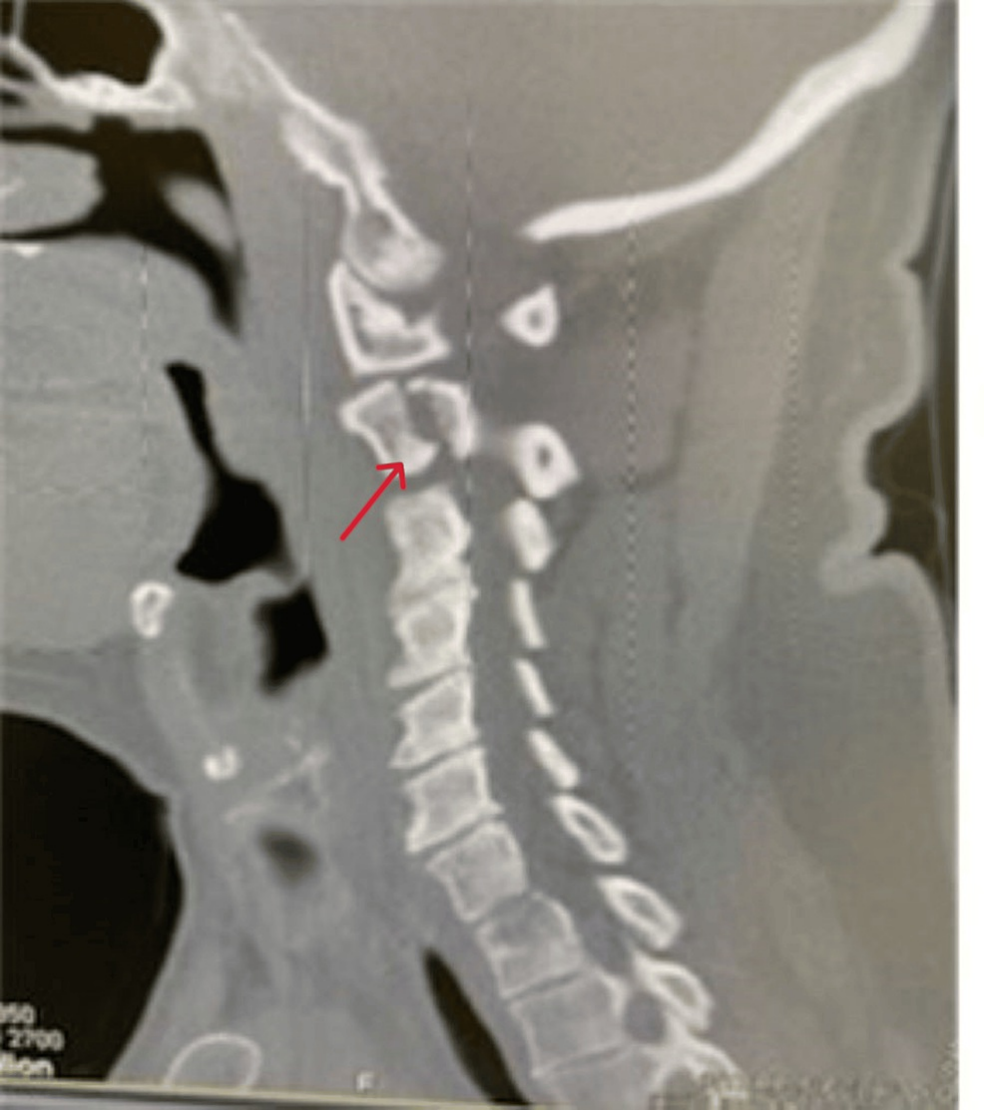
Non-contrast CT (sagittal view) of the cervical spine. Note the spondylolisthesis.
